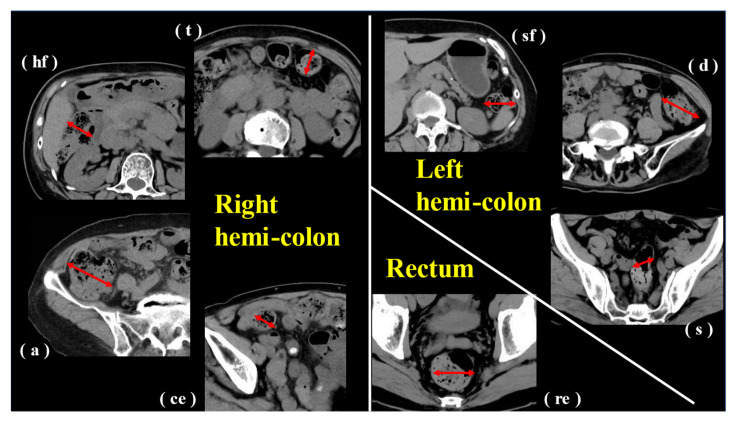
Simple CT image (axial image) of the abdomen and pelvis at each site of right hemi-colon, left hemi-colon and rectum. Diameter was measured at the most dilated site of the intestine (red line). (ce), cecum; (a), ascending colon; (hf), hepatic flexure; (t), transverse colon; (sf), splenic flexure; (d), descending colon; (s), sigmoid colon; (re), rectum.
